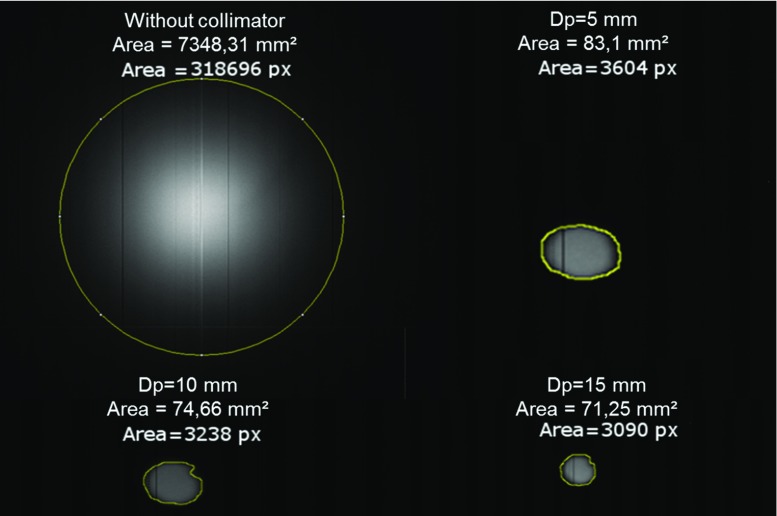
Change of the radiation zone, a without shielding b–d the change with shielding in variant distances (Dp) between outlet hole and X-ray tube, “Area” shows the size of radiation zone and number of pixels that are radiated
